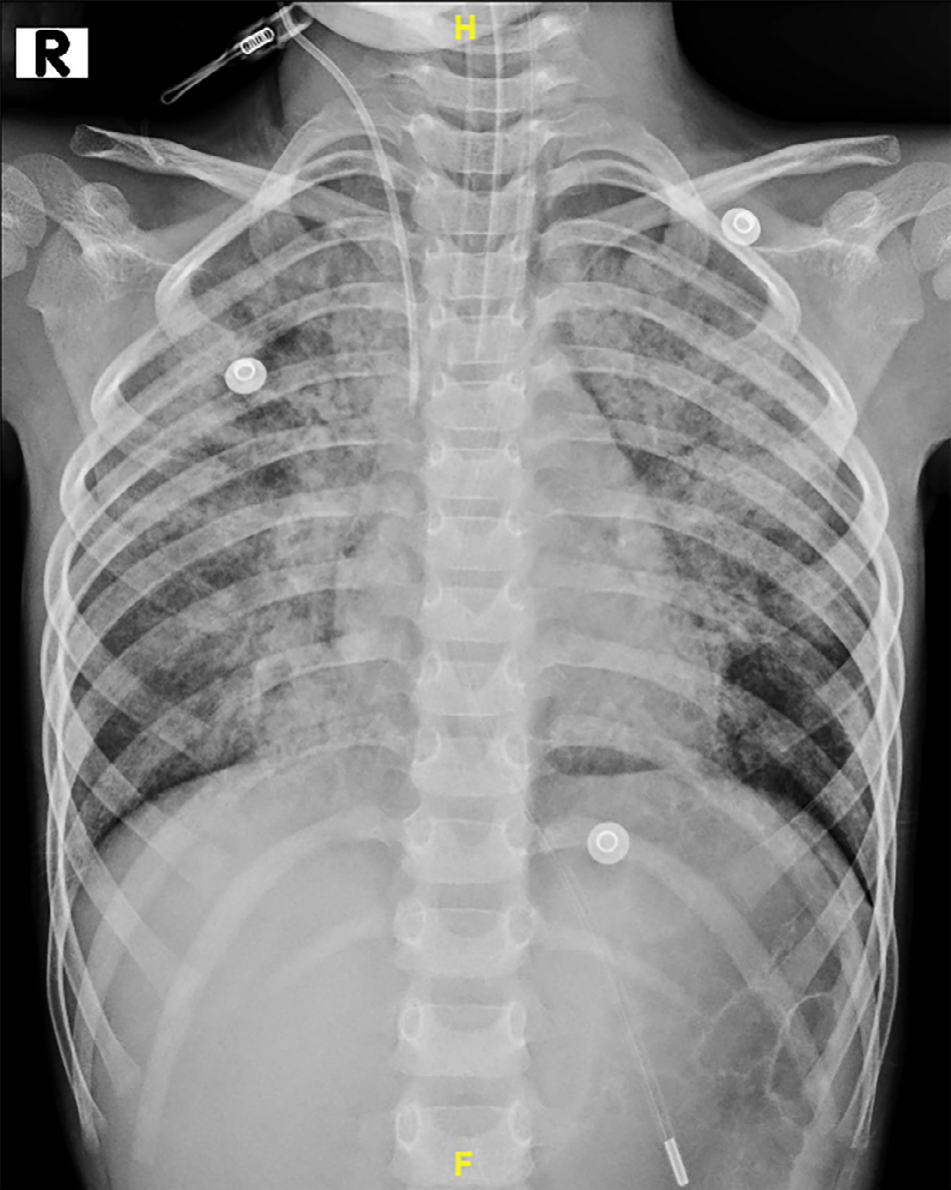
Chest radiograph (X-ray) showing bilateral diffuse nonhomogeneous opacities.
